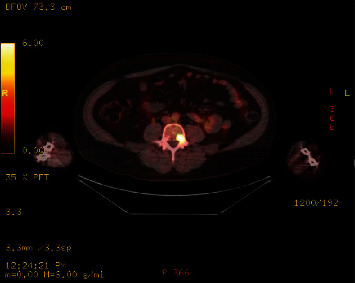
Increased uptake seen in the left L4 pedicle measuring 1.8 cm × 1.2 cm demonstrates SUV max of 6.8. This lesion is consistent with biopsy-proven osseous metastatic disease.
